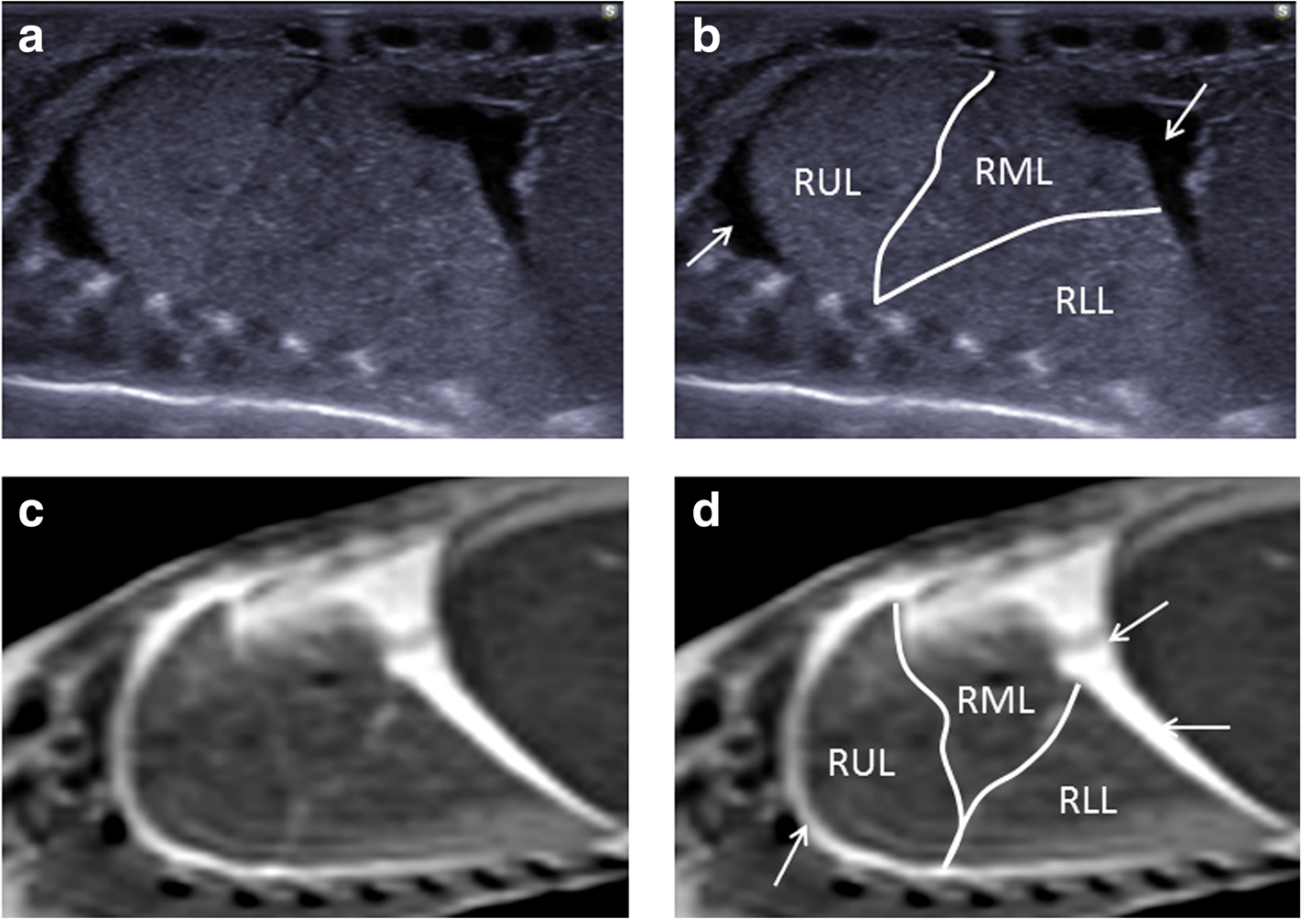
Paired sagittal postmortem ultrasound (a, b) and T2-weighted postmortem MRI images at 1.5 T (c, d) of the right lung in the same 18-week gestational-aged foetus, 12 days after death. These images clearly demonstrate the three different pulmonary lobes (RUL—right upper lobe, RML—right middle lobe, RLL—right lower lobe). The solid white arrows on the labelled images show normal expected physiological pleural fluid seen as part of postmortem change and should not be described as pathological
